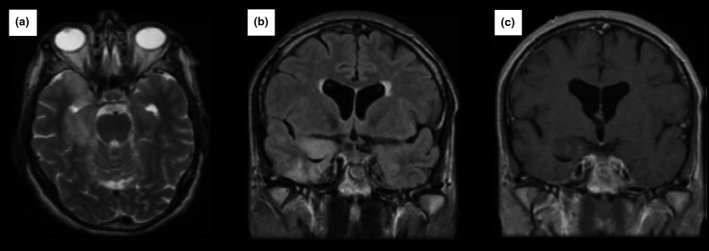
It is a 41‐year‐old man without no past medical history, admitted for generalized epilepticus status. *Brain MRI* shows on T2‐weighted images (a) and FLAIR sequences (b) hyperintensity in the right temporo‐insular without enhancement on gadolinium‐enhanced T1‐weighted images (c). Diagnosis of syphilitic LE was made after the biological investigations
